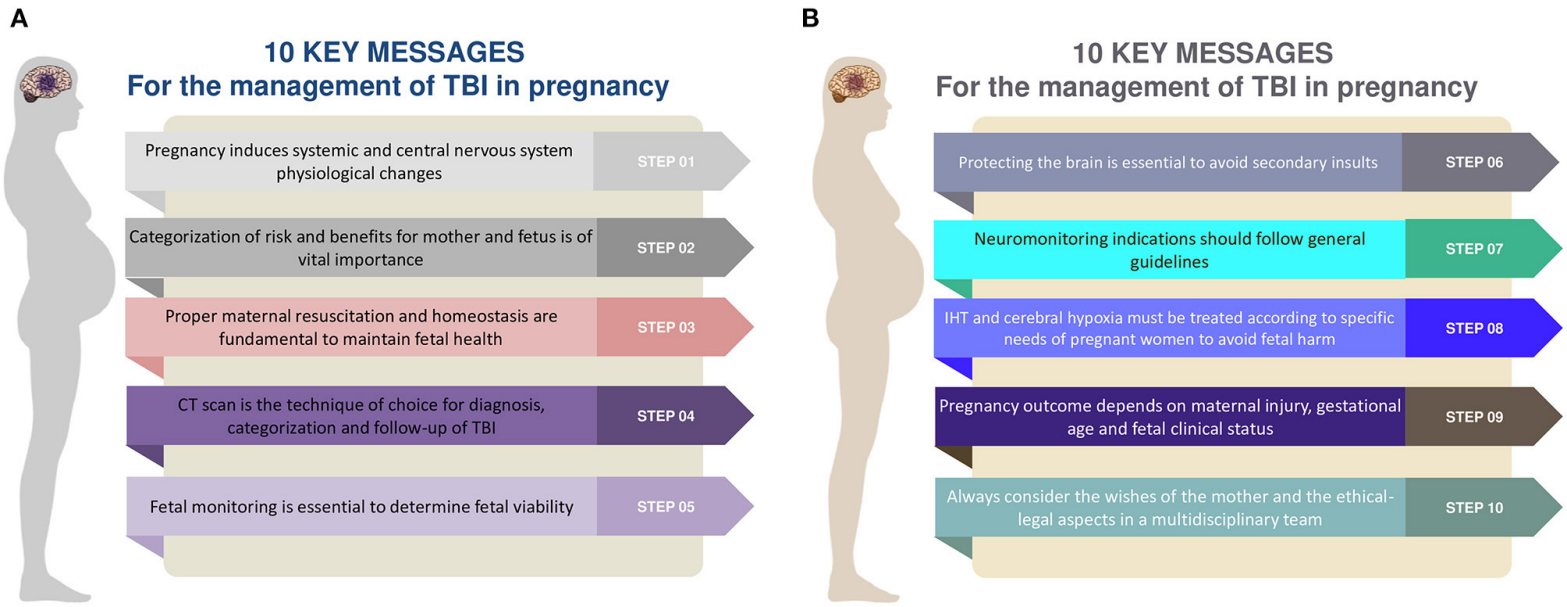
(A,B) Ten rules and steps for the management of traumatic brain injury in the pregnant woman. TBI, traumatic brain injury; CT, computed tomography; IHT, intracranial hypertension.
